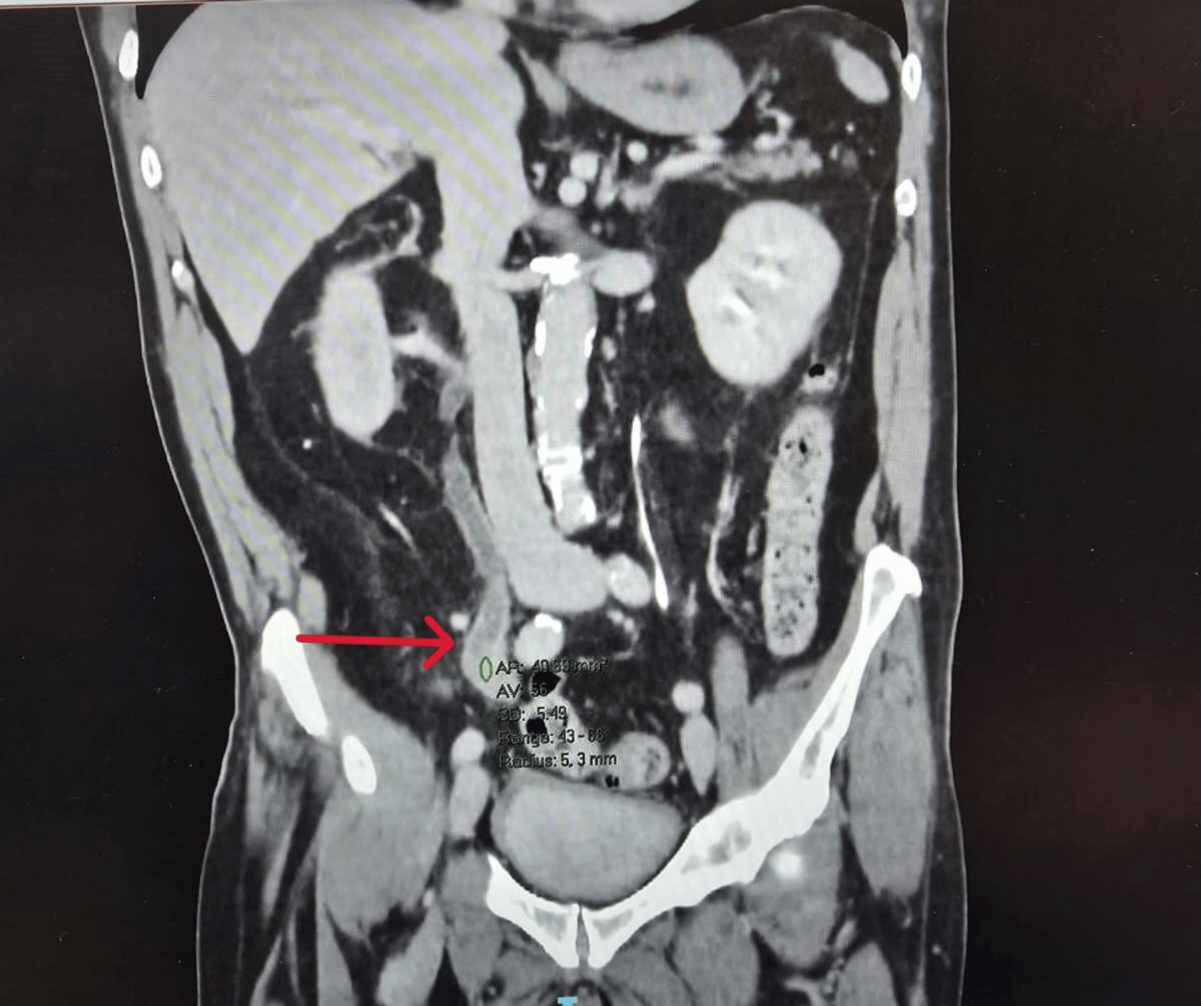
CT Urogram coronal view CT Urogram coronal view showing right hydroureter (Red Arrow) leading into right hydronephrosis.
